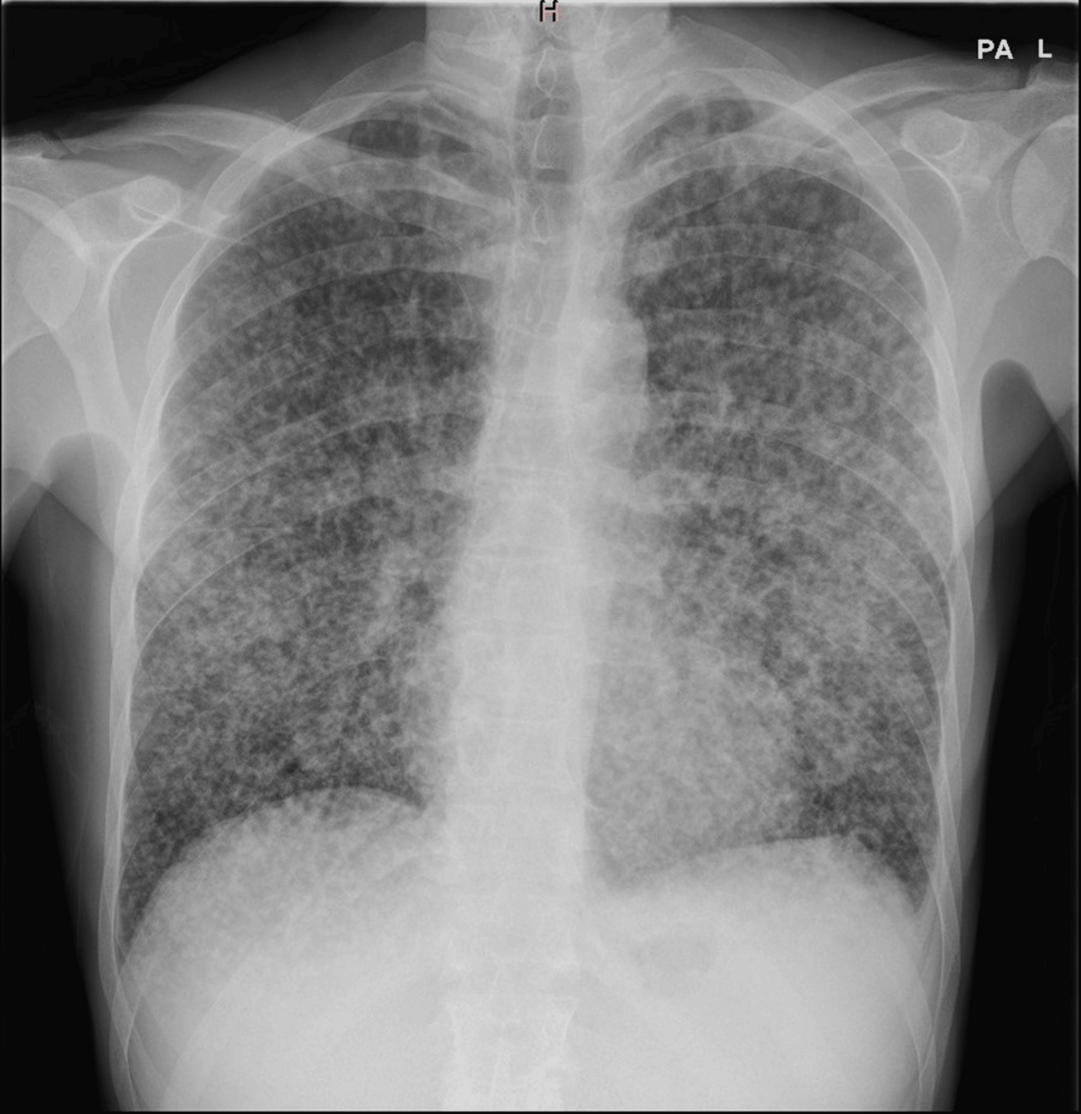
Chest X-ray of this patient showing disseminated tuberculosis
